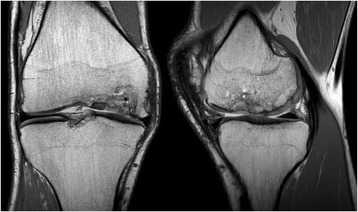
Post-Treatment PD weighted Coronal and Sagittal MRI imaging at 6 months indicating articular cartilage regeneration at the site of the osteochondral defect with smooth integration with the surrounding joint surface
